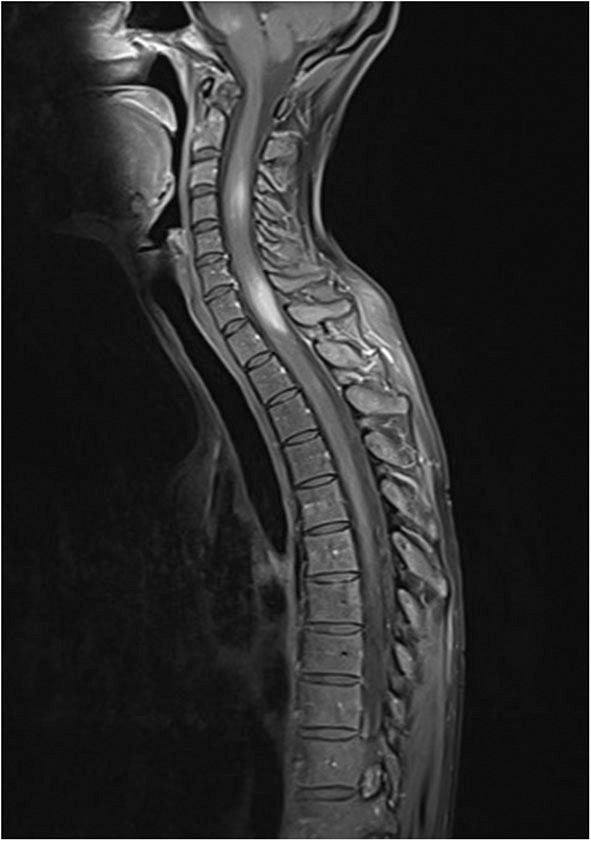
Hyperintense lesions in medulla oblongata and in cervical and upper thoracic spinal cord in contrast enhanced, fat suppressed T1 MRI technique.
